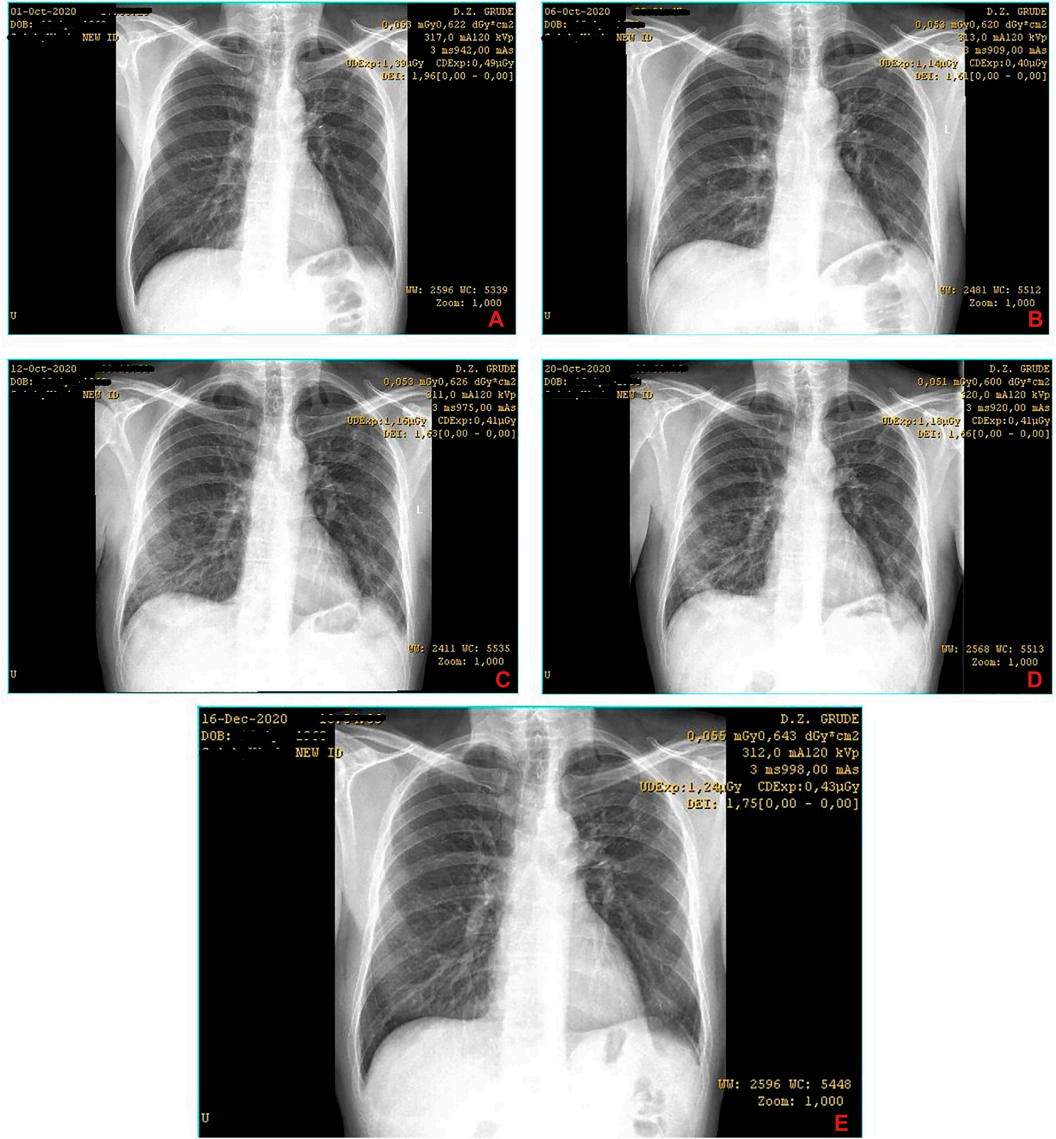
Series of chest X-ray images of COVID-19 patient from case 1. The images are chronologically* arranged in a–z order (the letter mark is indicated in the lower right corner of each image)^§^. *The dates of records are visible in the top right corner of each image. ^§^Identifiable patient’s data are covered.
